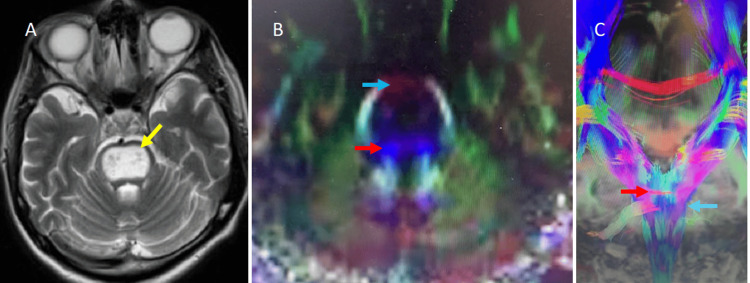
(A) Axial MRI hyperintense image shows a heterogeneous hyperintense lesion in the pons (yellow arrow). (B, C) The axial and coronal images with diffusion tensors of the pons show compression of corticospinal fibers in blue (blue arrows) and transverse pontine fibers in red (red arrows).
